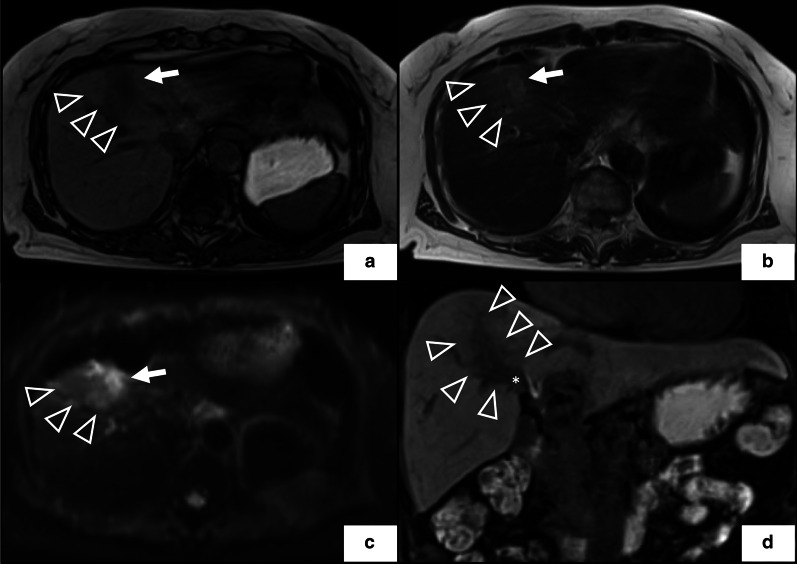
Preoperative magnetic resonance imaging findings. The tumor (arrow head) adjacent to the scar of radiofrequency ablation (arrow) exhibits low intensity on the T1-weighted image (a) and a slightly high intensity on T2- (b) and diffusion-weighted images (c). d Hepatobiliary phase of sagittal scan shows a low-intensity mass (asterisk) that continued from the tumor into the right hepatic duct
